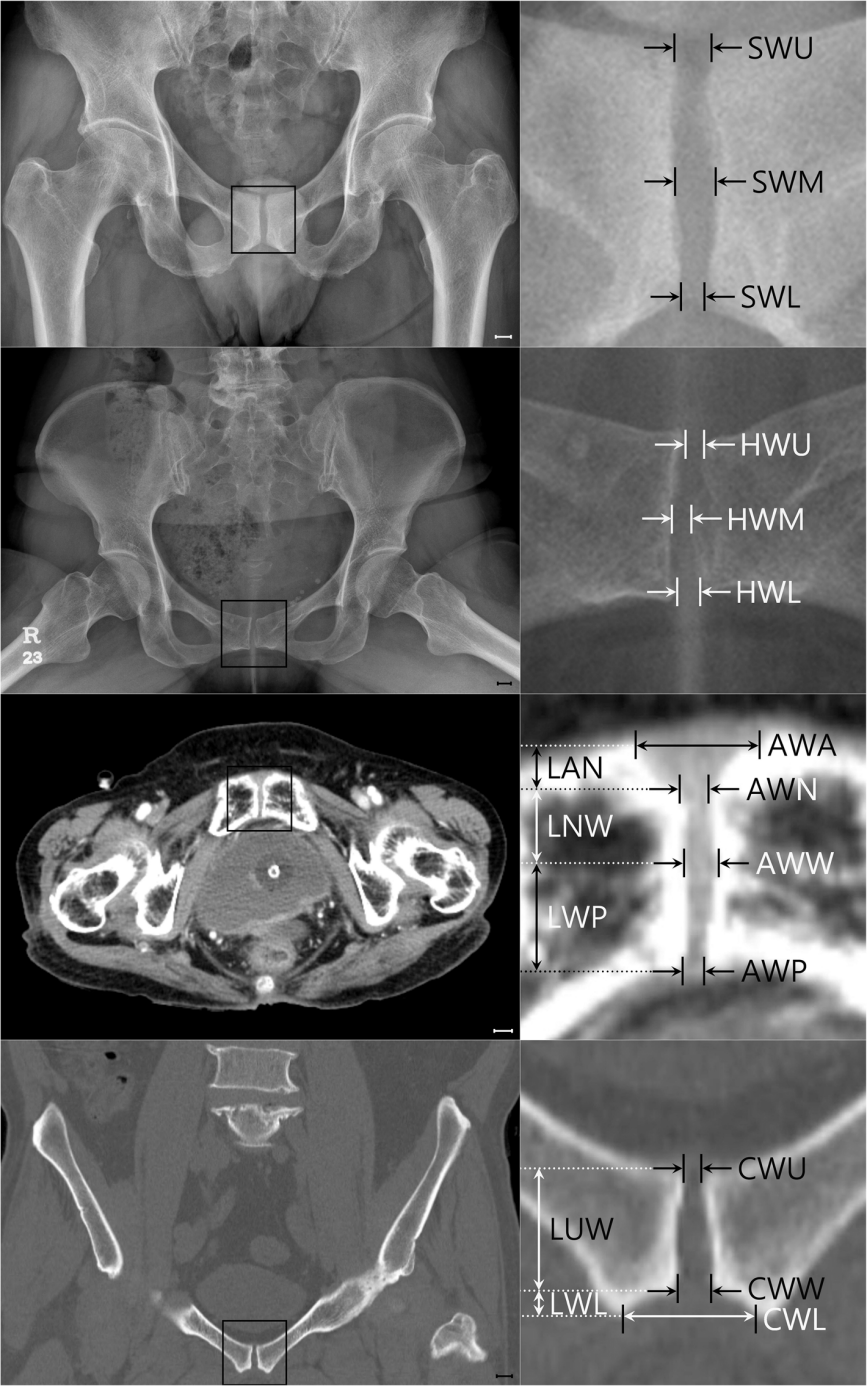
Measurement of the width of the pubic symphysis. First raw: pelvis AP (supine), second raw: pelvis AP (hip abduction view), third raw: pelvic CT (axial view), fourth raw: pelvic CT (coronal view). The scales in the left columns indicate 1 cm each
